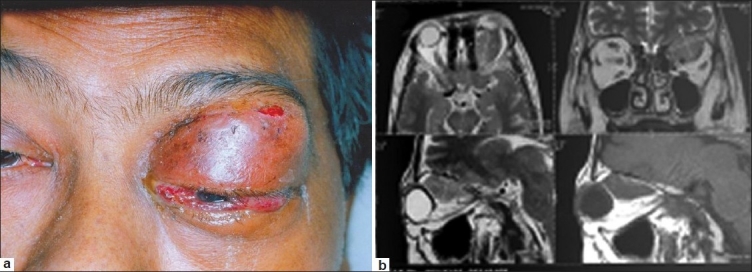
(a) Orbital mass at presentation (b) MRI findings
